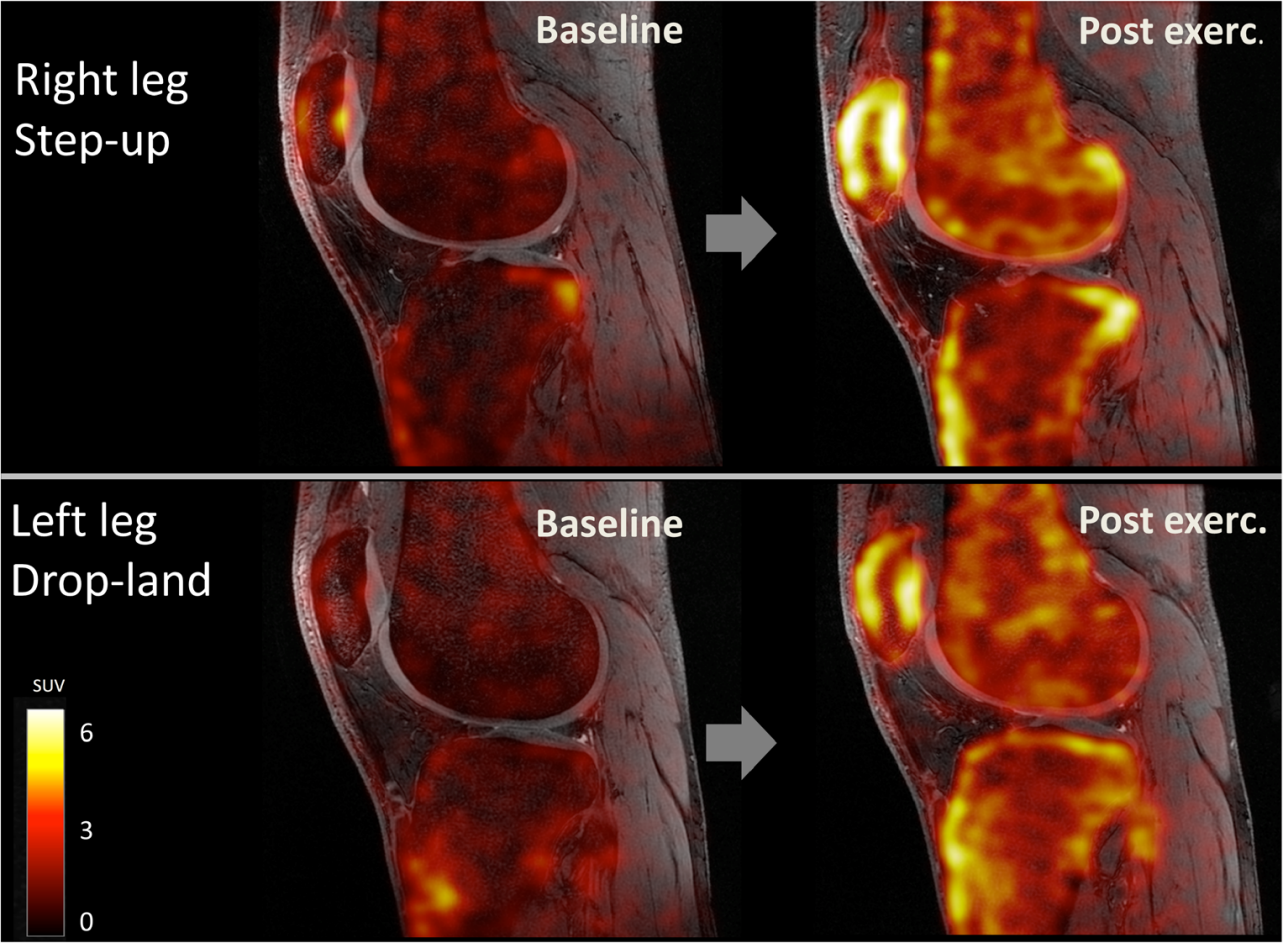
[^18^F]NaF SUV images for one subject at baseline and post exercise. Example images from each leg of one subject before and after exercise. SUV values increased significantly in all subjects after performing the one leg step-up (right leg) and one leg drop–land (left leg) exercise. SUV increases were higher in the step-up leg and varied regionally throughout the bone tissues of the knee (*p* < 0.05)
